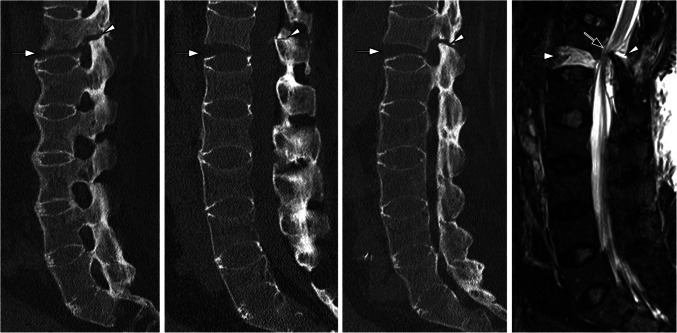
A patient with advanced axial spondyloarthritis and minor trauma. The patient presents with a vertebral column fracture at the D12 level (white arrow) accompanied by involvement of the posterior elements, as denoted by the white arrowheads, resulting in an unstable condition. Magnetic resonance imaging (MRI) reveals a corresponding spinal canal stenosis (black arrow) that is not clearly assessable with CT
